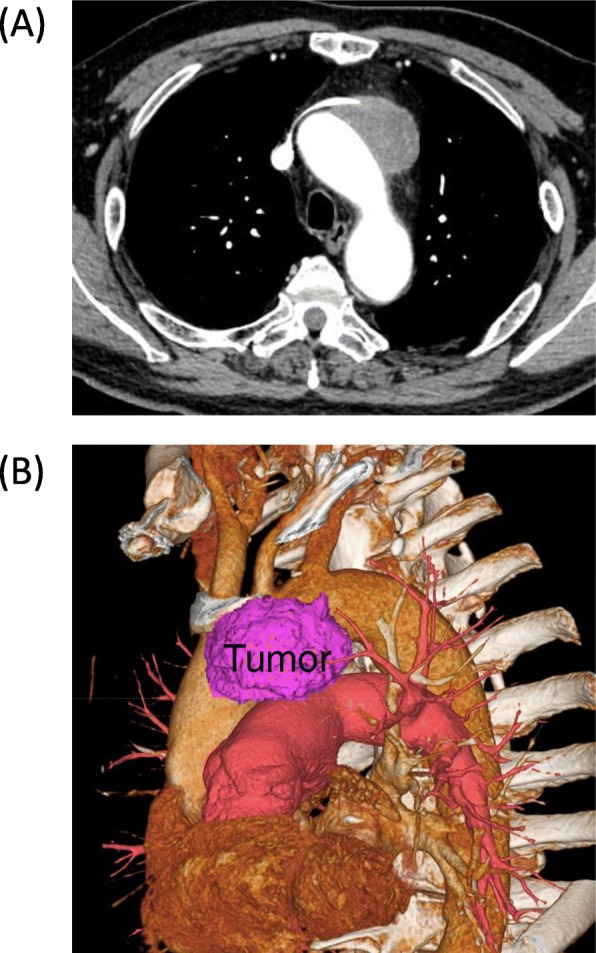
Preoperative radiographic images after chemotherapy. a Chest computed tomography image shows anterior mediastinal tumor, 55 × 55 × 50 mm, with AA invasion. b Three-dimensional image constructed using SYNAPSE VINCENT® (Fuji Film, Tokyo, Japan). AA, aortic arch; CT, computed tomography
